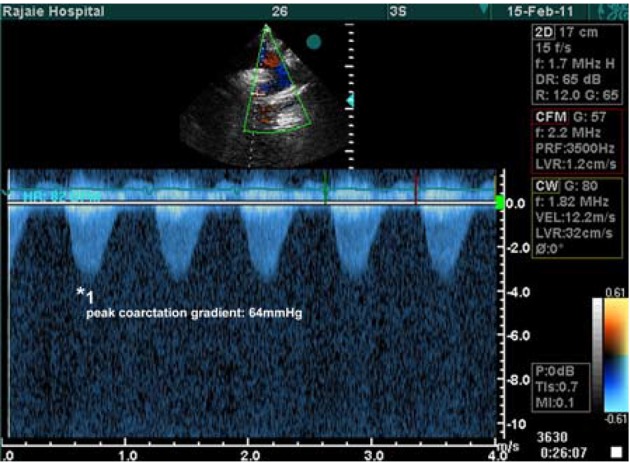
Continuous Wave Doppler in Coarctation Site
